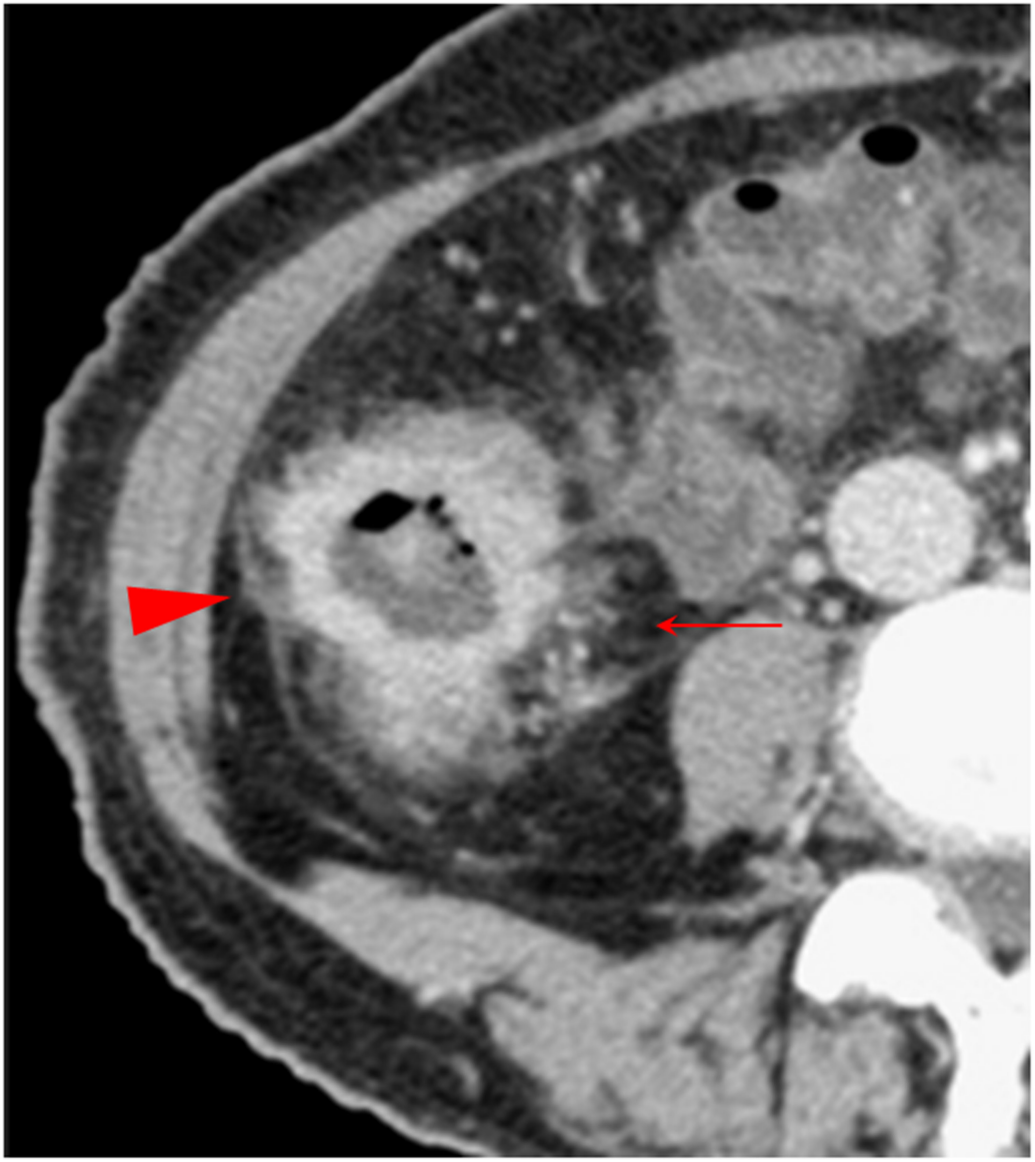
Combination of multiple CT signs. The transverse section of a middle ascending colon adenocarcinoma, pT4bN1Mx, in a 73-year-old woman is shown. The extensive reticulonodular fat stranding (solid arrow), fascia thickening, the fascia adhesion and concave, extra-fascial nodules, and streaks (arrowhead) are shown. The pericolic fat and the extra-fascial fat exhibit diverse increased attenuation patterns. Thickened lateral conal fascia with adhesion and a concave contour toward tumor is shown.
